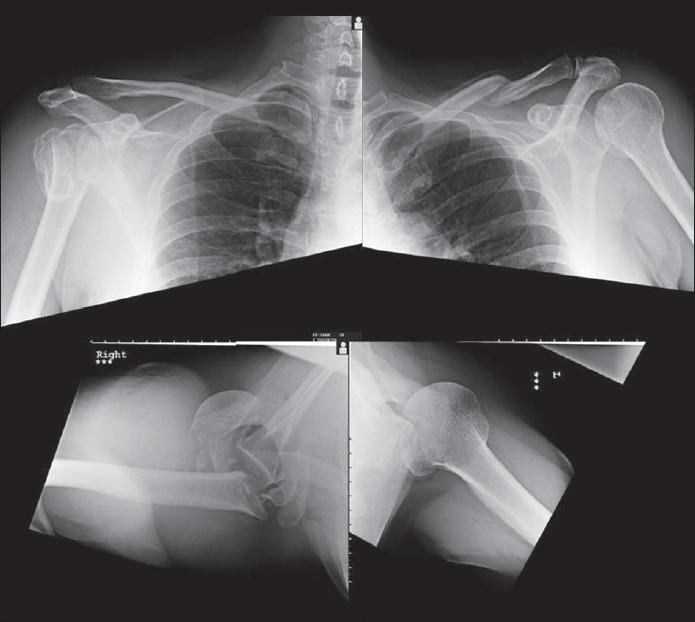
Preoperative radiograph of both shoulders
